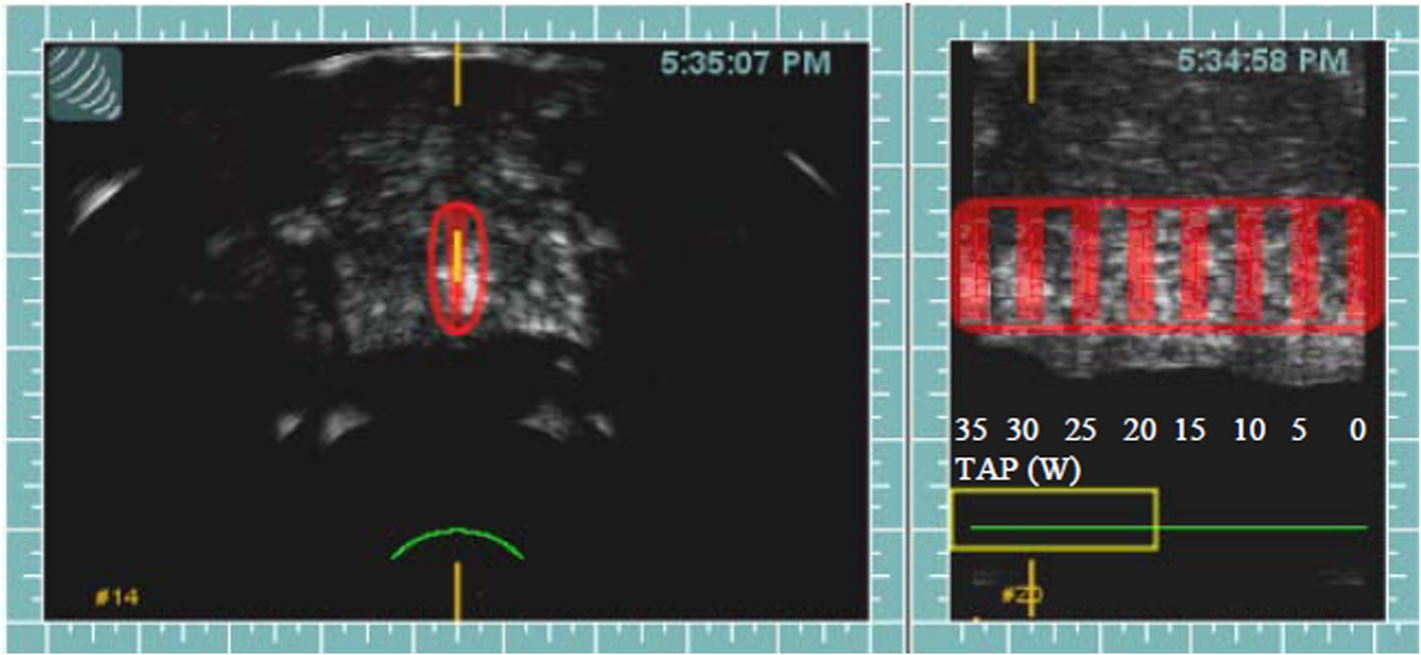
Sonablate HIFU ablation planning on chicken tissue (before TCM color coding). The left and right panel images are B-mode ultrasound transverse and sagittal image of the tissue. Each red color box is a HIFU ablation site. The HIFU ablation started from the right and the transducer was translated to the left in 6 mm increments, and the acoustic power was increased by 5 watts for each subsequent exposure
